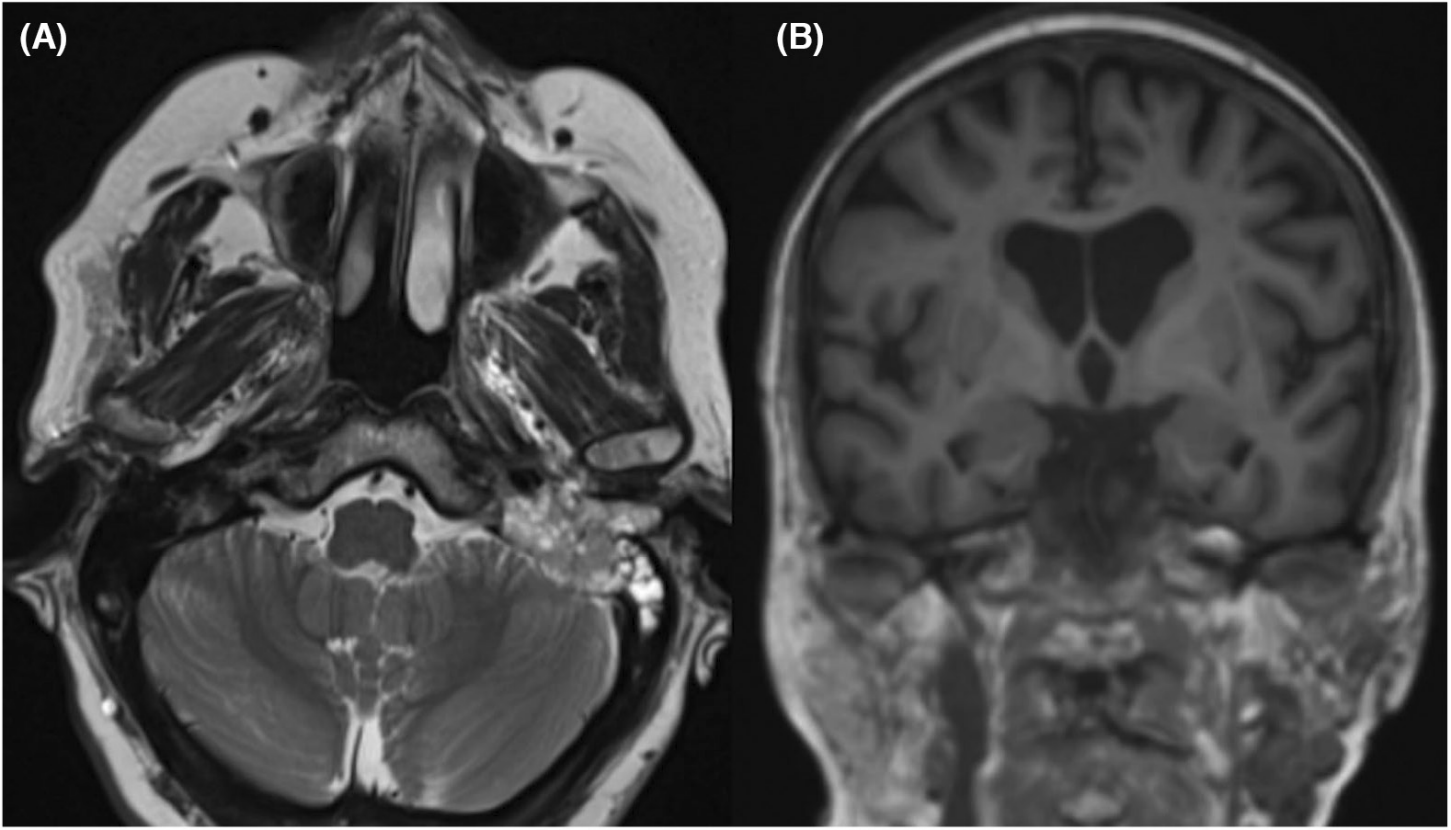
Pre‐operative CT Scans; (A) Axial CT Section; the tumor involved the left pontocerebellar angle; (B) Coronal CT Section; the neoplasm involved the left petrous bone, the left pontocerebellar angle and the fibers of VII, VIII, IX, X, XI, XII cranial nerves.
